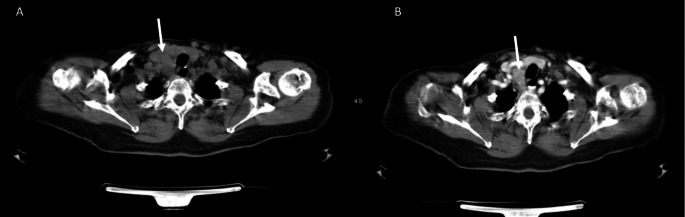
CT scan of the neck. The arrows show the presence of a parathyroid gland nodule of 2 × 3.3 cm in the right paratracheal region with extension to the superior mediastinum. (A) before and (B) after contrast agent
